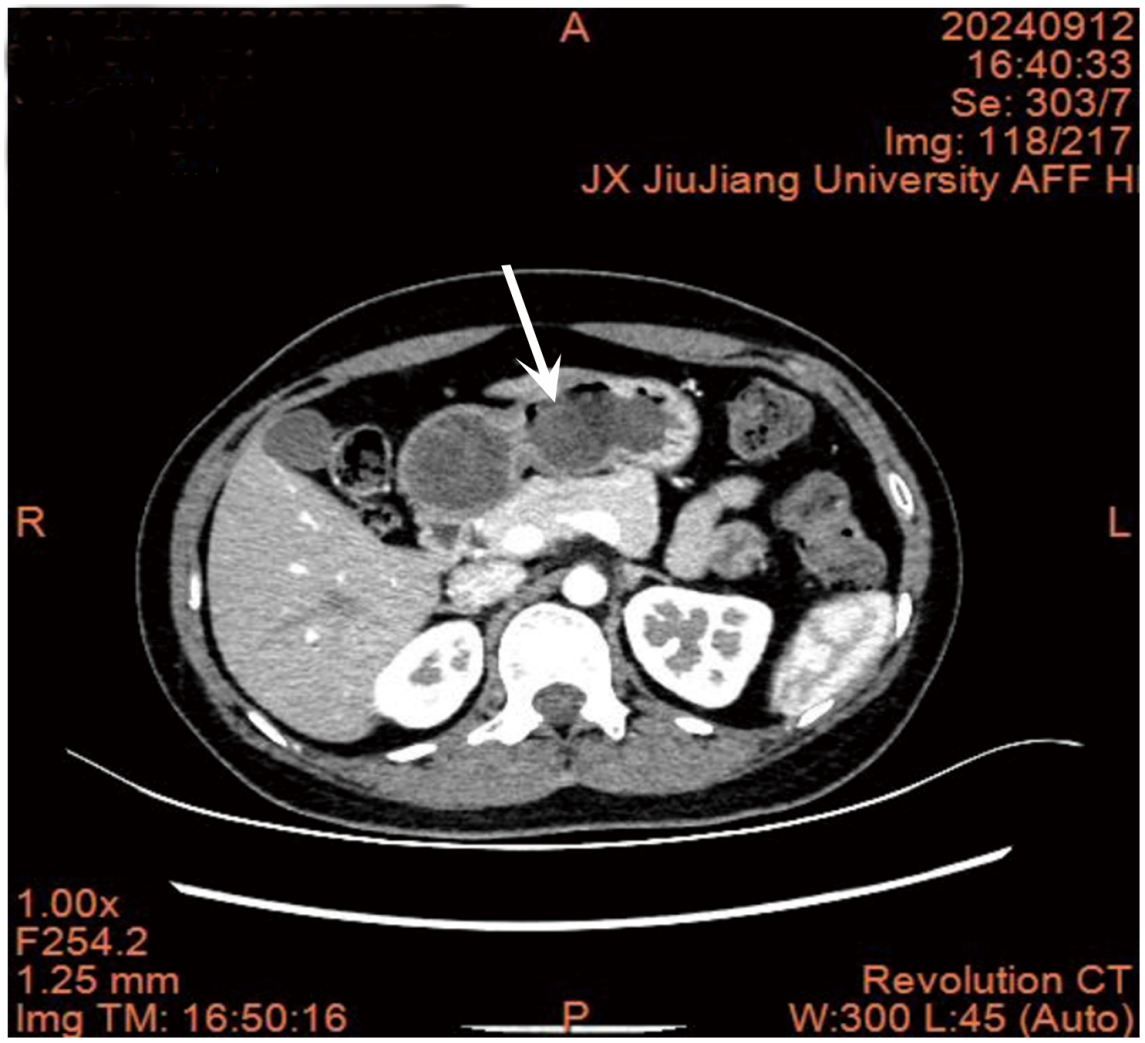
Abdominal CT scan results.
